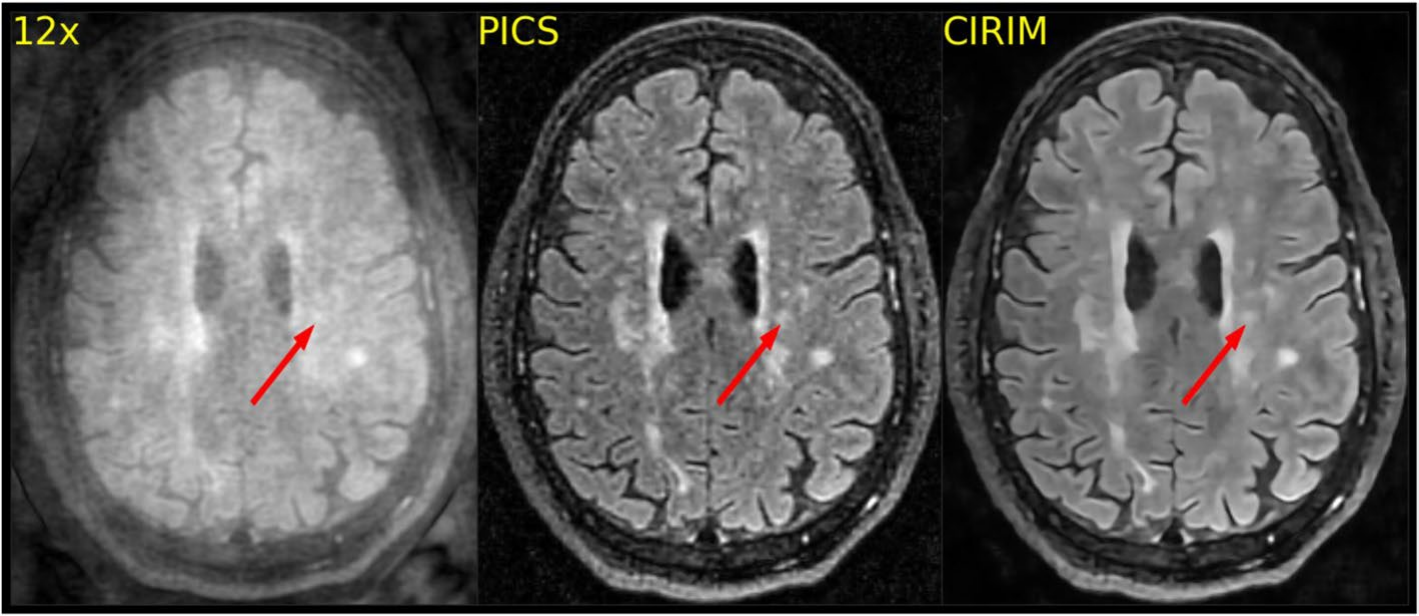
Reconstructions of a 12 times accelerated FLAIR scan, where the CIRIM yielded a more blurred reconstruction than PICS did for small T_2_ high signal intensities among patients with small vessel disease (example indicated by the arrow)
